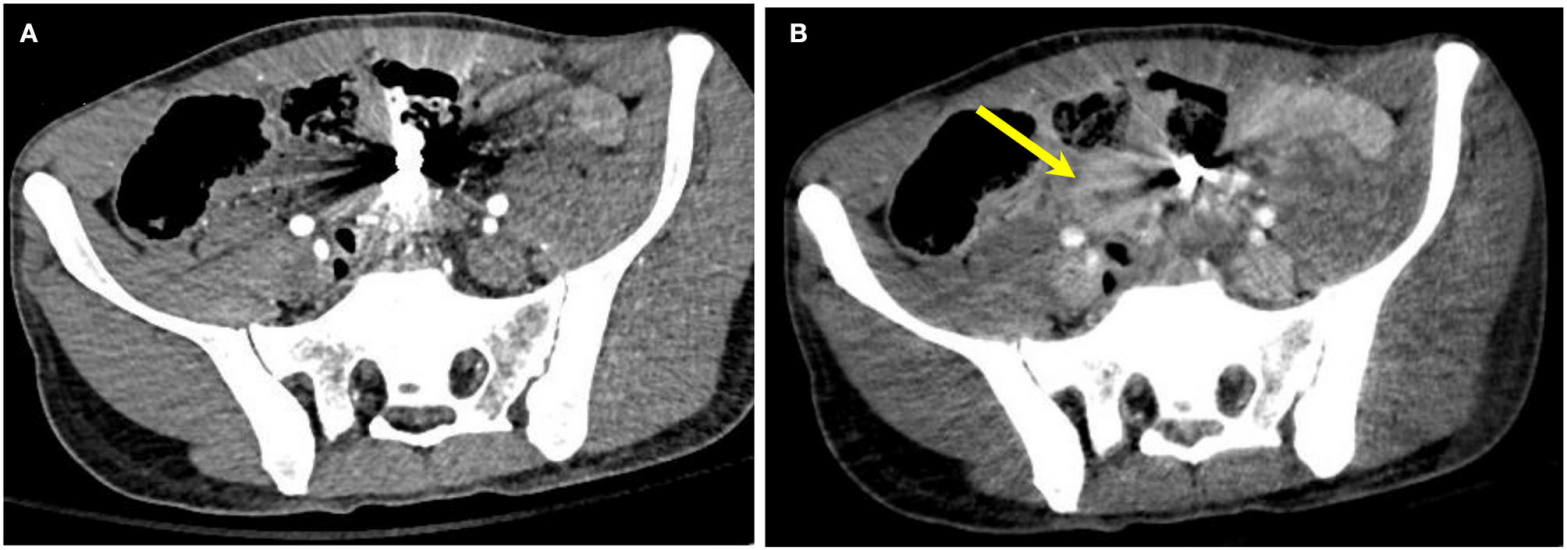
A 25-year-old man with a gunshot wound. (A) Conventional CT shows bullet fragments with streak artifacts. (B) Image 140 keV demonstrates decreased streak artifact revealing thickened bowel loops (arrow).
